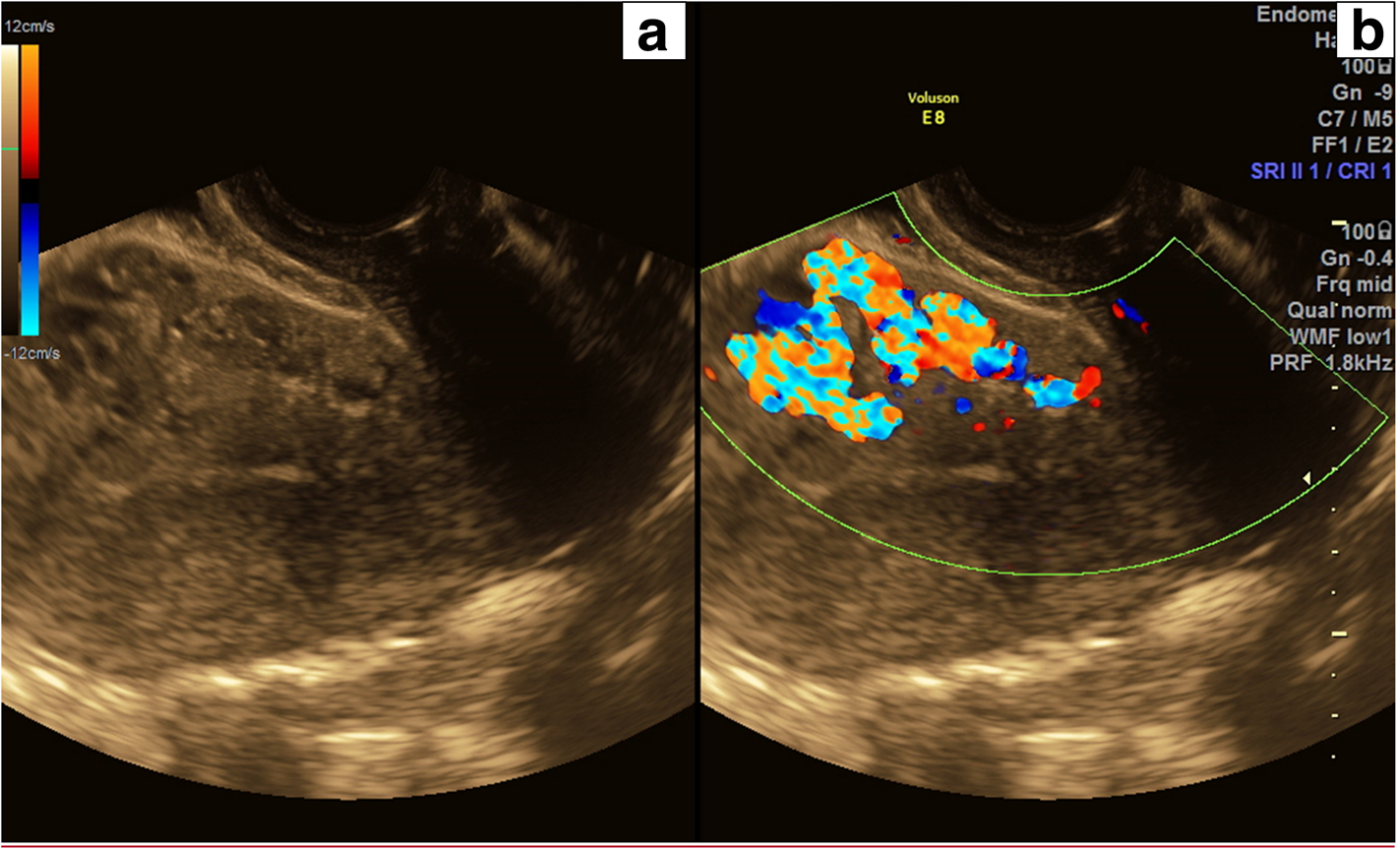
a Sagittal transvaginal pelvic ultrasound image demonstrating a serpinginous hypoechoic area in the anterior myometrium compatible with an anterior cornual AVM. b Colour Doppler overlay demonstrating markedly increased high-flow vascularity of the AVM
